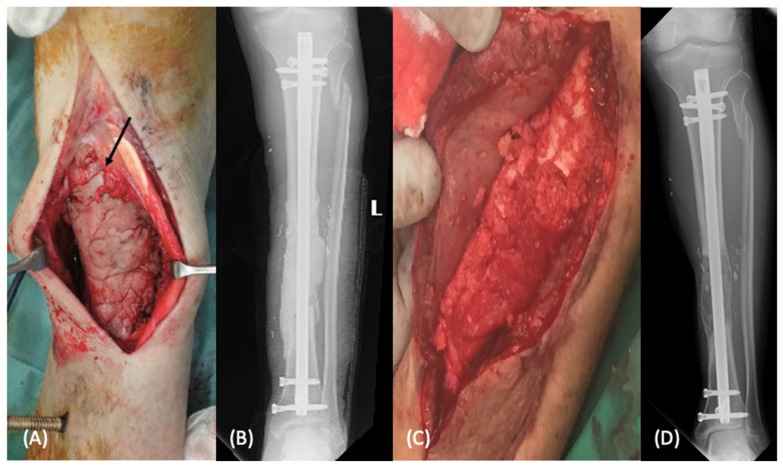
Masquelet technique for diaphyseal tibial defect demonstrated in intraoperative photos of the antibiotic spacer placement (A) with post-operative radiographs (B). The patient underwent subsequent second stage autologous bone grafting into induced membrane (C) with post-operative radiographs seen in image (D).
